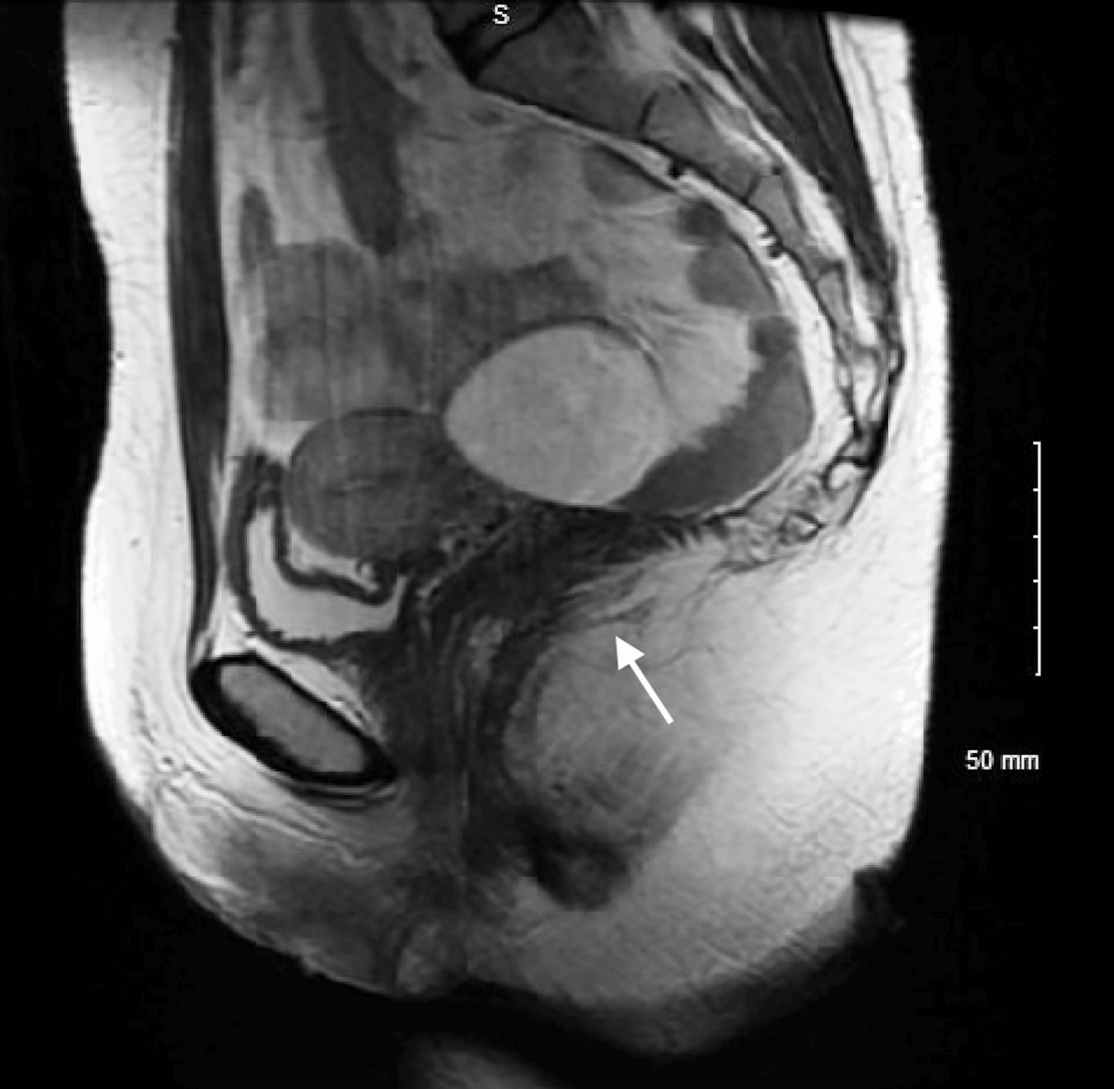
Pelvic magnetic resonance imaging (MRI) post-panproctocolectomy in a 28-year-old woman with Crohn's disease. Sagittal MRI of a female pelvis following panproctocolectomy, showing absent rectum and sigmoid colon. A linear high-signal tract extending posteriorly from the vaginal vault indicates a fistula formation.
